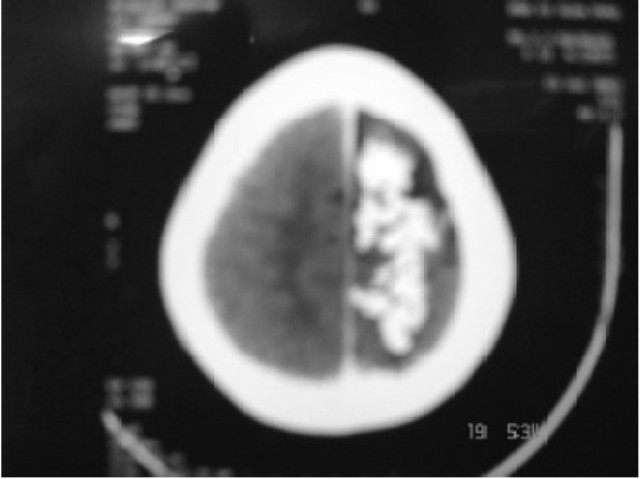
CT scan of brain showing left parietal angioma with dystrophic cerebral calcification
